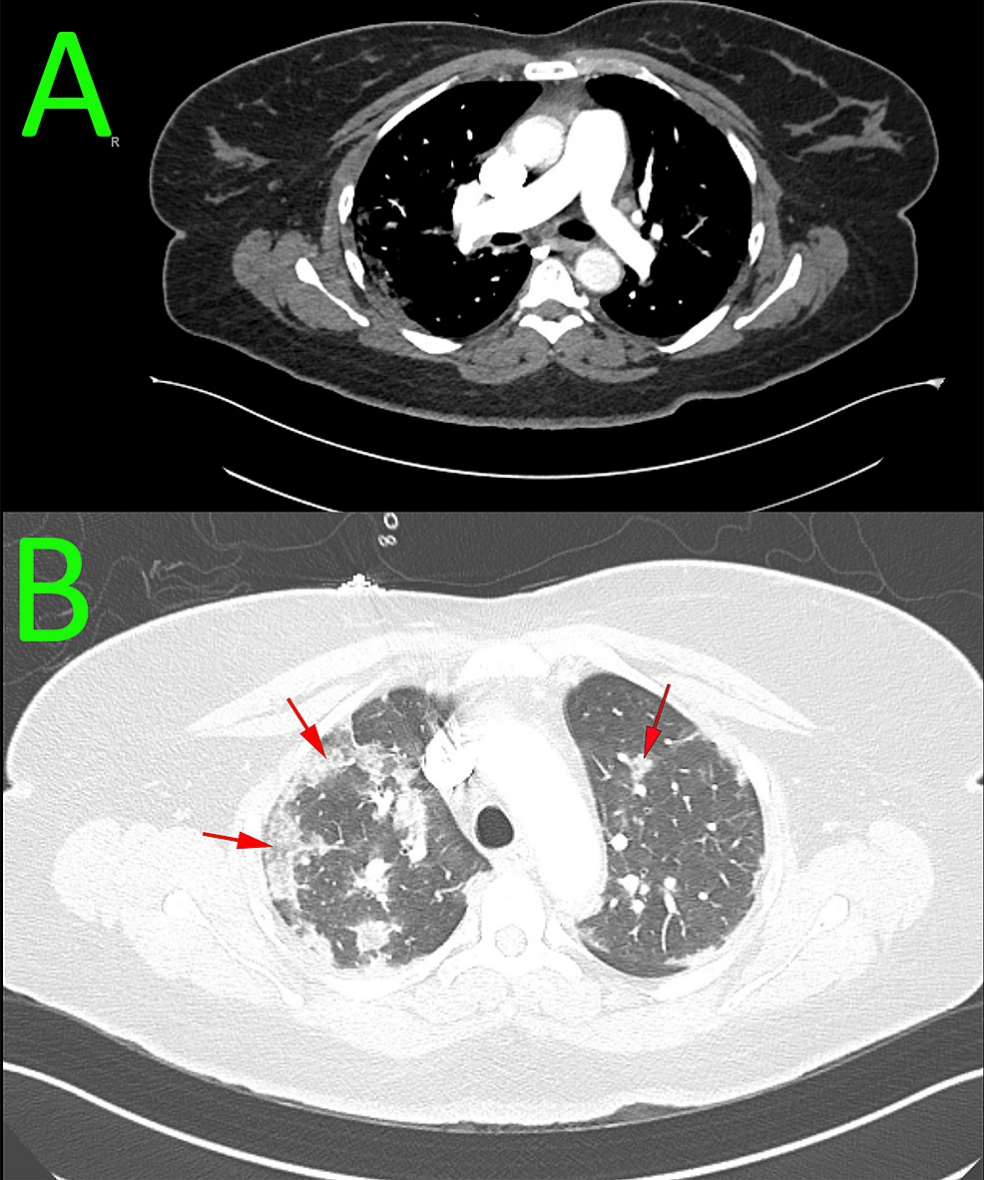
CT angiogram of the chest A: the image revealed no filling defect seen in the central or segmental pulmonary arterial branches to suggest pulmonary thromboembolism. B: the image demonstrated moderate bilateral patchy peripheral ground-glass opacities (red arrows) CT: computed tomography
